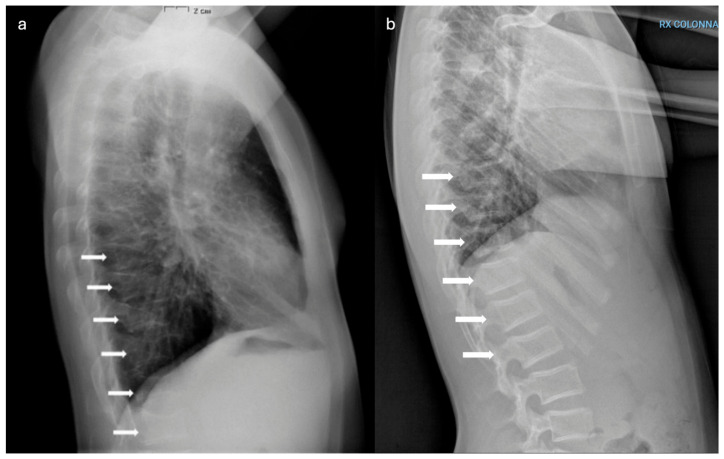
Comparative lateral spine radiographs of the mother (a) and son (b). Images demonstrate multiple compression fractures as highlighted by the arrows, and kyphosis in the mother (a), compared with mild endplate deformities at T9–T12 in the son and early superior endplate deformities in L1–L2, as highlighted by the arrows (b).
